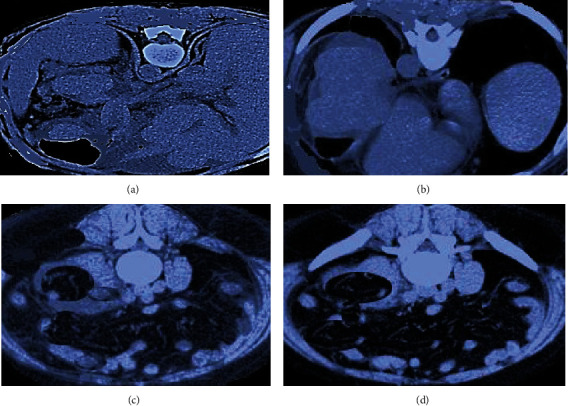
CT image of cirrhotic effusion in a 48-year-old male patient. (a) and (b) were plain CT images; (c) and (d) were enhanced CT images. Red arrow marked ascites.
